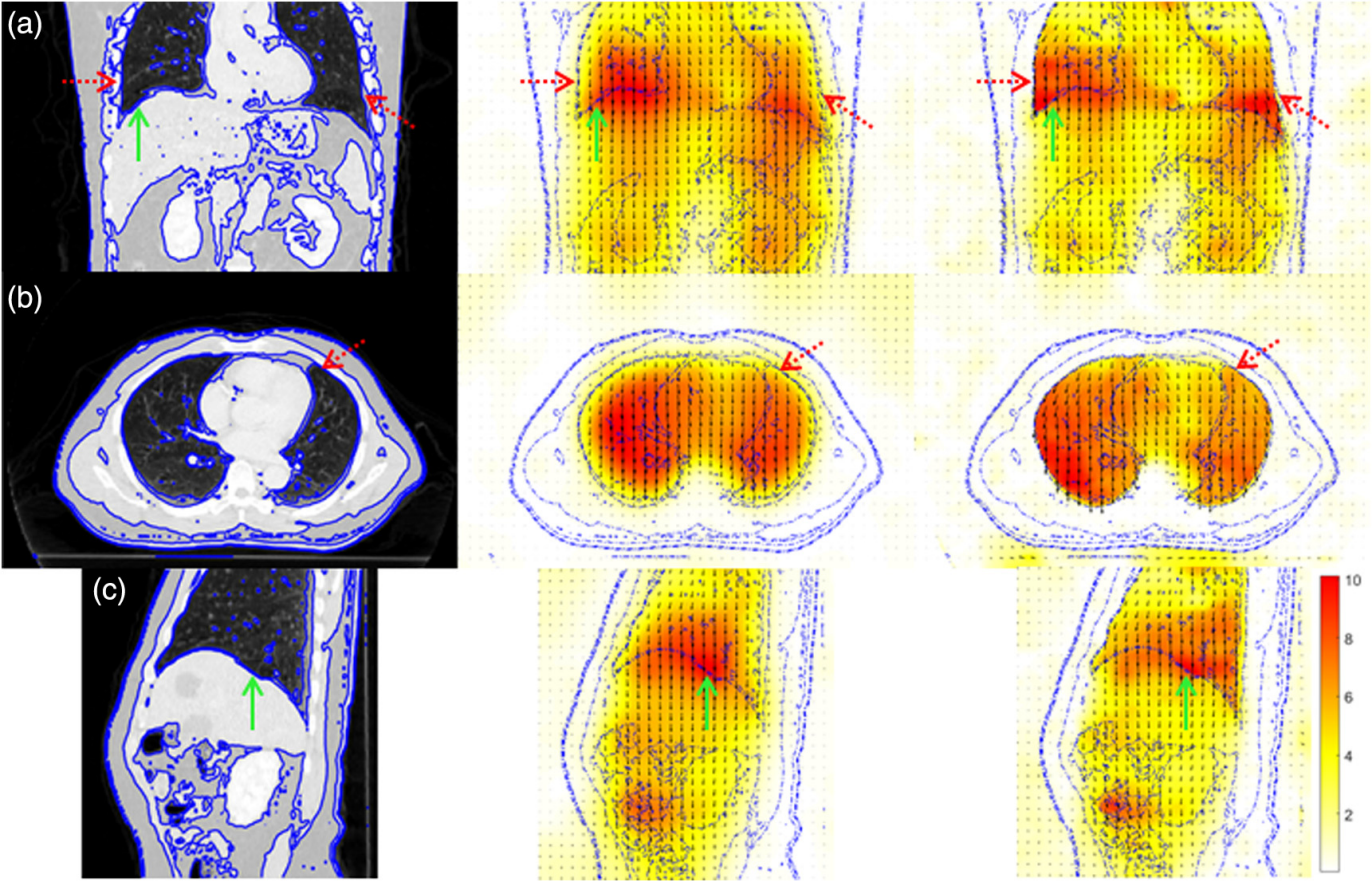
Main anatomical views of resulting 3-D displacement fields for case #P0 of the liver CT dataset: (a) coronal, (b) axial, and (c) sagittal views for the color-coded magnitude of the displacement field estimated using Demons with isotropic Gaussian kernel, iso-dem, (middle) and guided image filtering with random SLIC clustering, rdn-gif, (right). (left) The reference image with the corresponding blue contour is shown for a guidance to the displacement field. Registration using our method (right) produces a visually smooth displacement field inside the lungs and liver, and at the same, estimates sliding motion at the lung and liver interface [depicted by corresponding red dotted (for lungs) and green solid (for liver) arrows].
